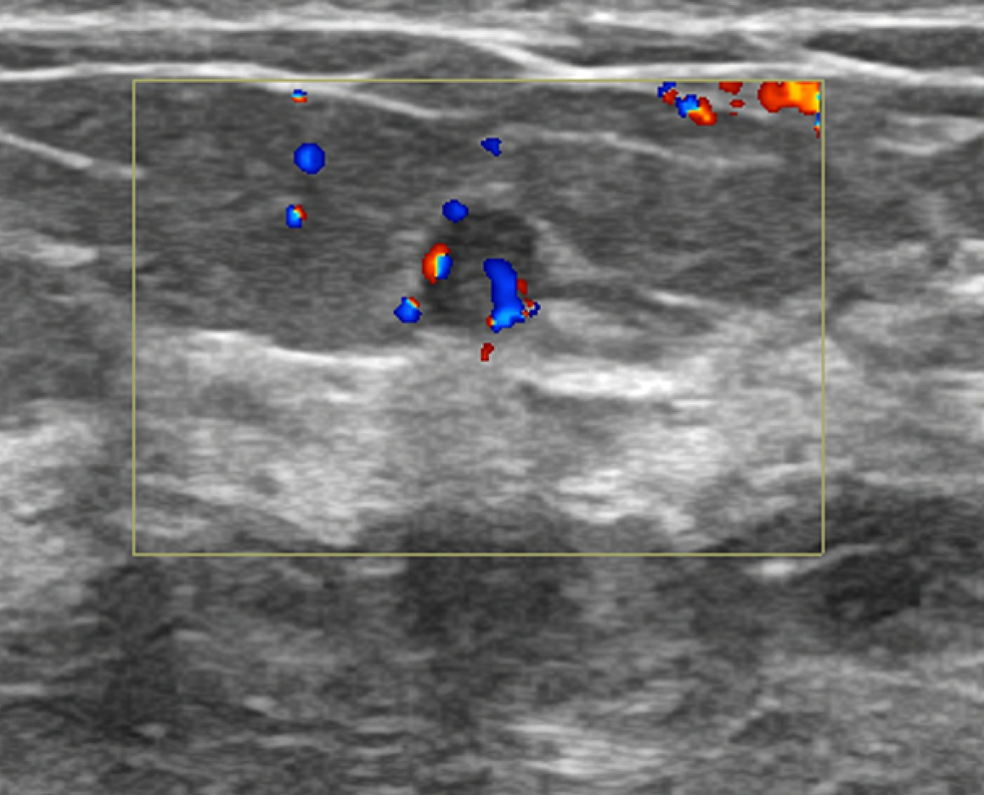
Ultrasound image of the breast demonstrating a hypoechoic solid lesion with posterior shadowing and associated internal vascularity
